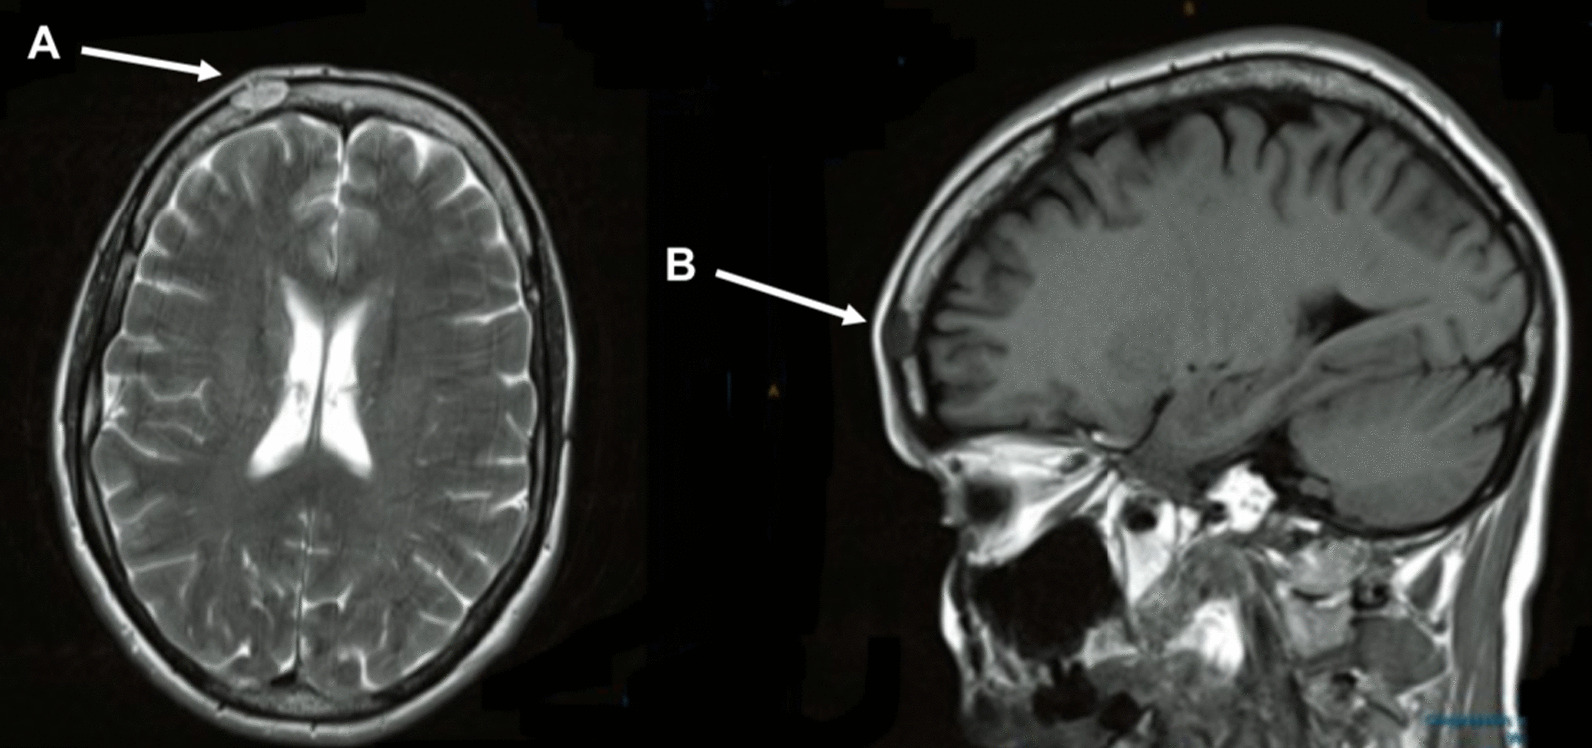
Head magnetic resonance imaging. a Axial T2 and b sagittal fluid-attenuated inversion recovery images. Both images demonstrate an oval 1.5-cm well-defined intradiploic lesion in the right frontal bone with a breach in the outer table of the skull overlying the lesion. The arrows denote the presence of the lytic lesion seen on magnetic resonance imaging
